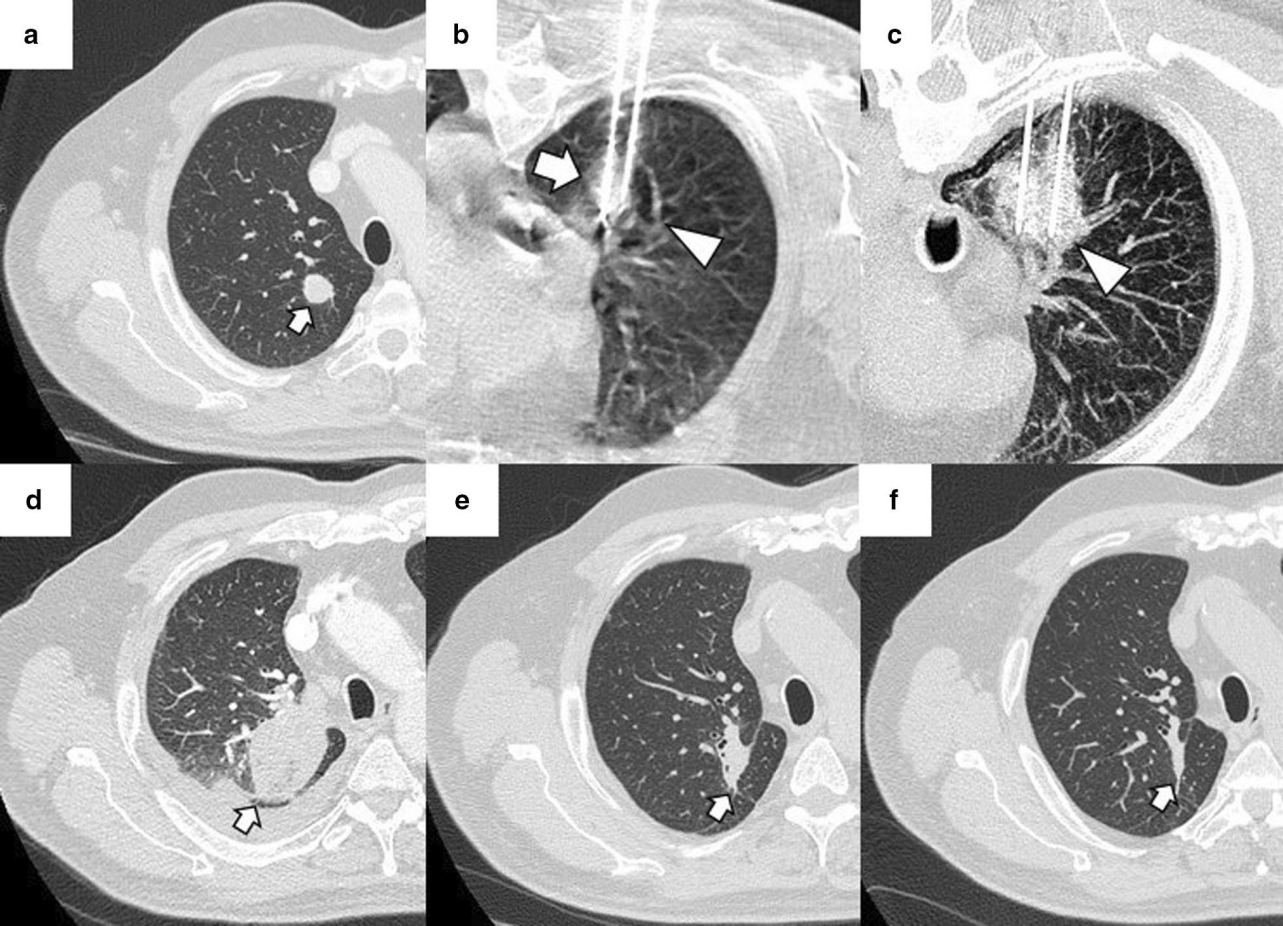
"Iceball" formation and further evolution to an area of lung hemorrhage in an image-guided percutaneous pulmonary cryoablation. a CT scan performed before ablative treatment, demonstrating a single metastatic lesion from a colon carcinoma in the upper right lobe (arrow). b, c CT-guided CA performed with two straight probes. Note the position of the probes, parallel and at the edges of the lesion. In (b) the ice ball (triangle) is observed surrounding the tumor (arrow). This ice ball was subsequently replaced by a ground-glass halo in (c) (triangle). d Contrast-enhanced CT scan performed one month after treatment, with a treated area more extensive than the original lesion (arrow). e, f The treated area gradually decreases in size at three (e) and six months (f) after treatment, consistent with successful treatment (arrows)
